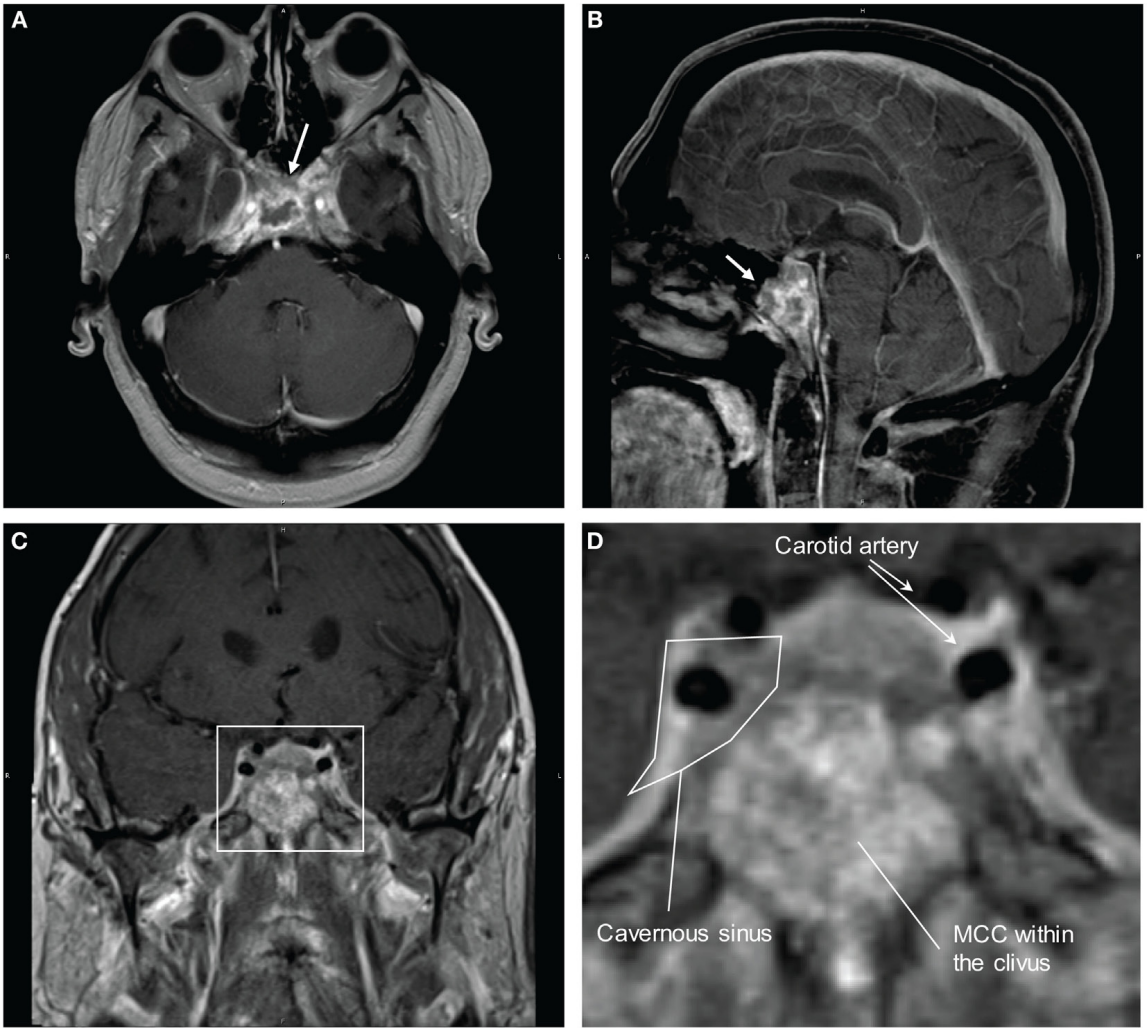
MRI brain with contrast showing Merkel cell carcinoma (MCC) metastasis to the clivus and invasion into the cavernous sinus. (A) Transverse view. Arrow is pointing to the mass in the clivus. (B) Sagittal view. Arrow is pointing to the mass in the clivus. (C) Coronal view. (D) Magnified view of the cavernous sinus from (C). There is invasion of the metastatic MCC into the cavernous sinus with likely impingement on the cranial nerves.
